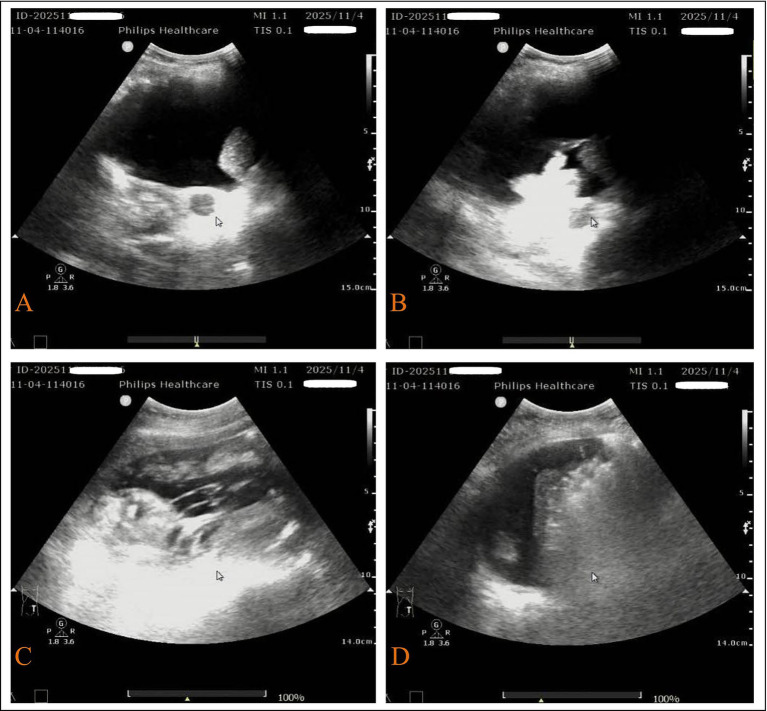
Intraoperative e-FAST imaging of pleuroperitoneal effusion. (A) Left chest: large pleural effusion. (B) Right chest: large pleural effusion. (C) Right perinephric region: fluid accumulation with nephrostomy tube visualized. (D) Abdomen: moderate ascites. e-FAST, extended focused assessment with sonography for trauma. All image captures preserved original metadata including essential information and dates, while patient names and institutional details were redacted in compliance with privacy protection protocols.
